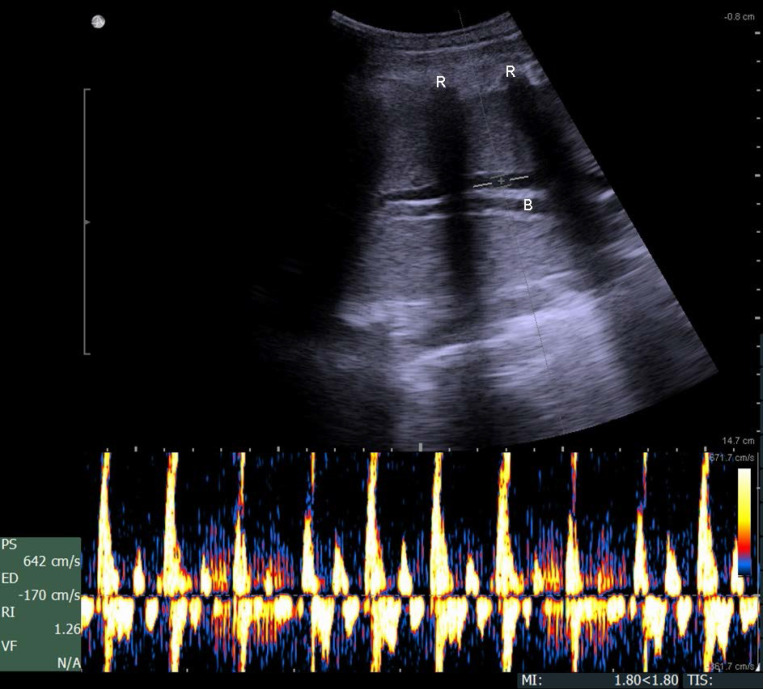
Duplex sonography imaging shows a biphasic oscillating flow in the pulmonary artery within the flooded lung (B, bronchus; R, ribs).
